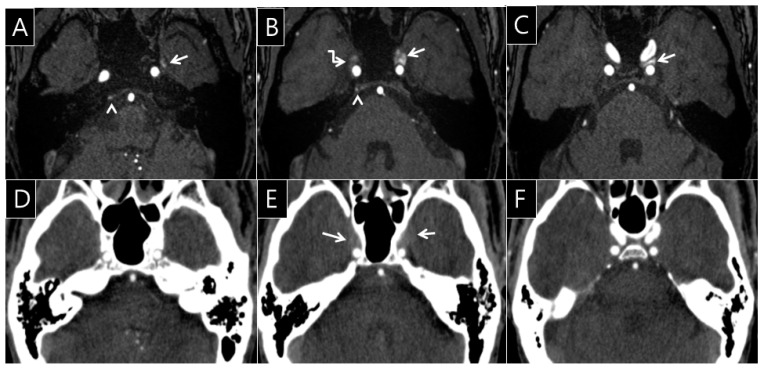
A 56-year-old man’s brain computed tomography angiography (CTA) and time of flight magnetic resonance angiography (TOF-MRA) images. On the TOF-MRA source images (A–C), the left pterygoid plexus (arrow, A) shows FRSI on TOF-MRA (A). The FRSI is contiguous into the left CS, occupying more than one-third of the CS on TOF-MRA (arrows, B, C, grade IV). Mild FRSI was observed in the right inferior petrosal sinus (arrowhead, A, B, grade I) and right CS (broken arrow, B, grade III). However, the opacification of the venous sinus is less than or equal to the density of pons (D, F, grade 0). The degree of opacification in the both CS is greater than the density of the pons in the same regions on brain CTA (arrows, E, grade I). Minimal agreement with the consensus score (*κ* = 0.30, 95% confidence interval: 0.10–0.51) is shown; Note: κ, degree of intra-observer agreement between CTA and TOF-MRA.
